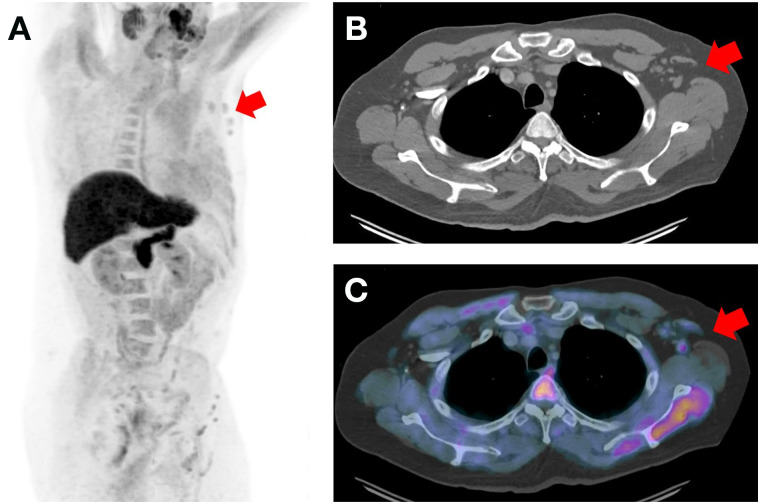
(A) ^18^F-fluciclovine PET maximum-intensity projection revealed 4 foci of unexpected avidity in left axilla (arrow). Remainder of uptake was physiologic. (B and C) Axial CT (B) and axial ^18^F-fluciclovine PET/CT (C) images show avidity localizing 4 mildly enlarged but morphologically normal lymph nodes (arrows). Remaining images (not shown) confirmed no other sites of potentially pathologic radiotracer avidity.
